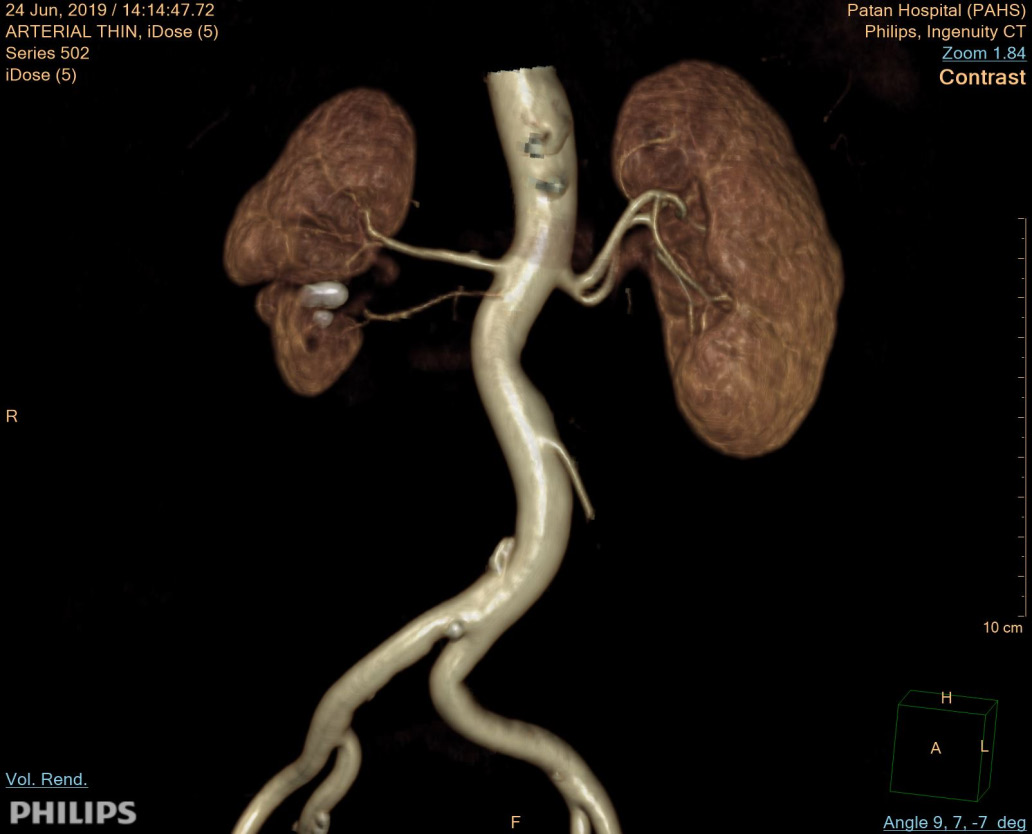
3D reconstruction of CT urography image in arterial phase showed a separate arterial supply to right supernumerary kidney from abdominal aorta and early segmental branching of left renal artery. Two large calculi in the fused right supernumerary kidney were also appreciated.
